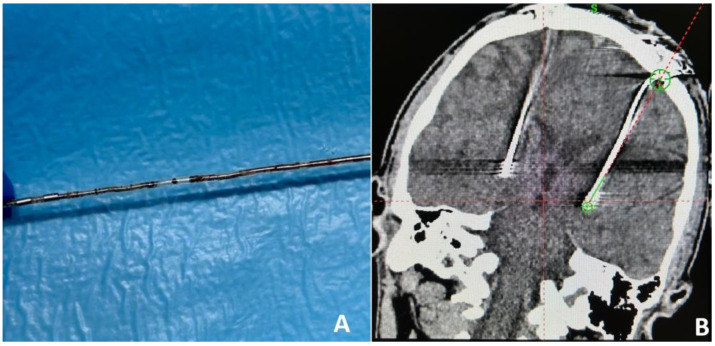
Intracranial extension wire fracture (A). Post-operative frontal CT after intracranial electrode replacement (B).
